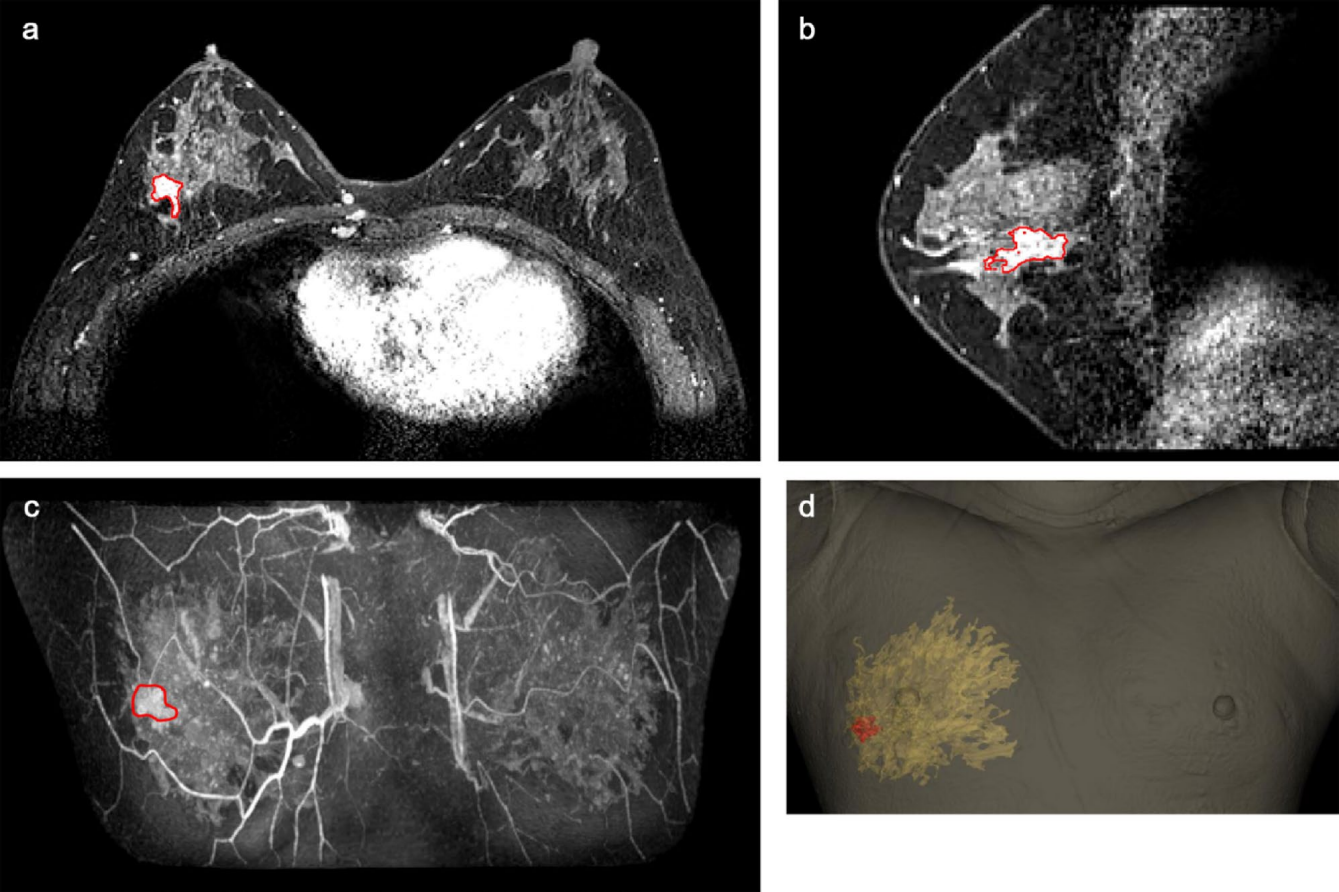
Segmentation of normal tissues and tumors in breast MRI. (A, B) Tumor segmentation in prone MRI. (C) Tumor segmentation in supine MRI. (D) 3D modeled breast and tumor based on prone/supine MRI.
